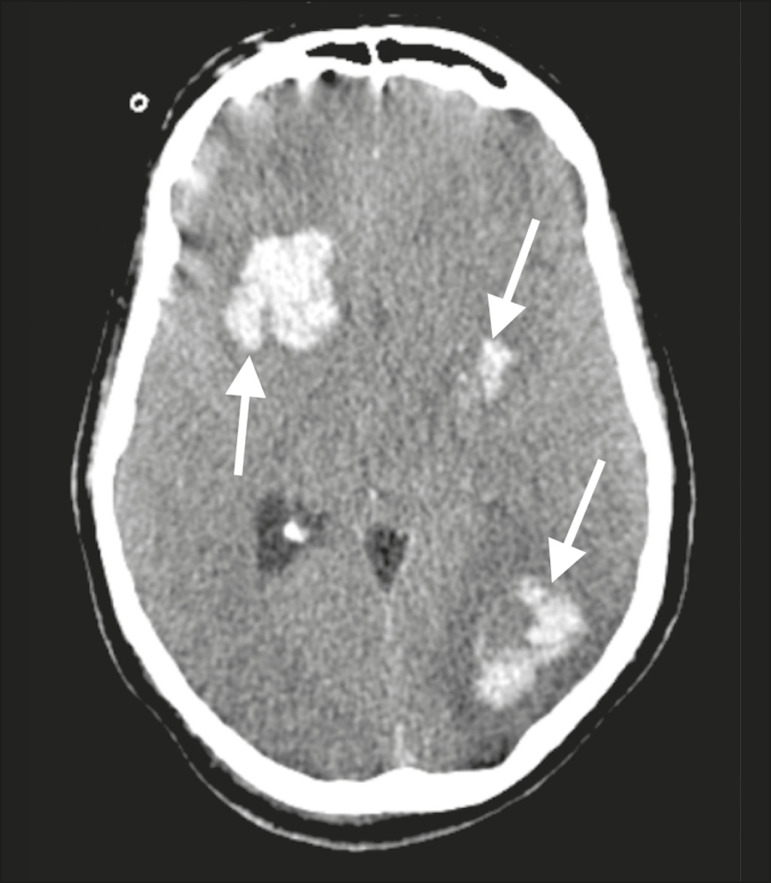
Case 5. Brain CT of a 49-year-old man with COVID-19 showing multiple lobar and basal ganglia hematomas (arrows).
